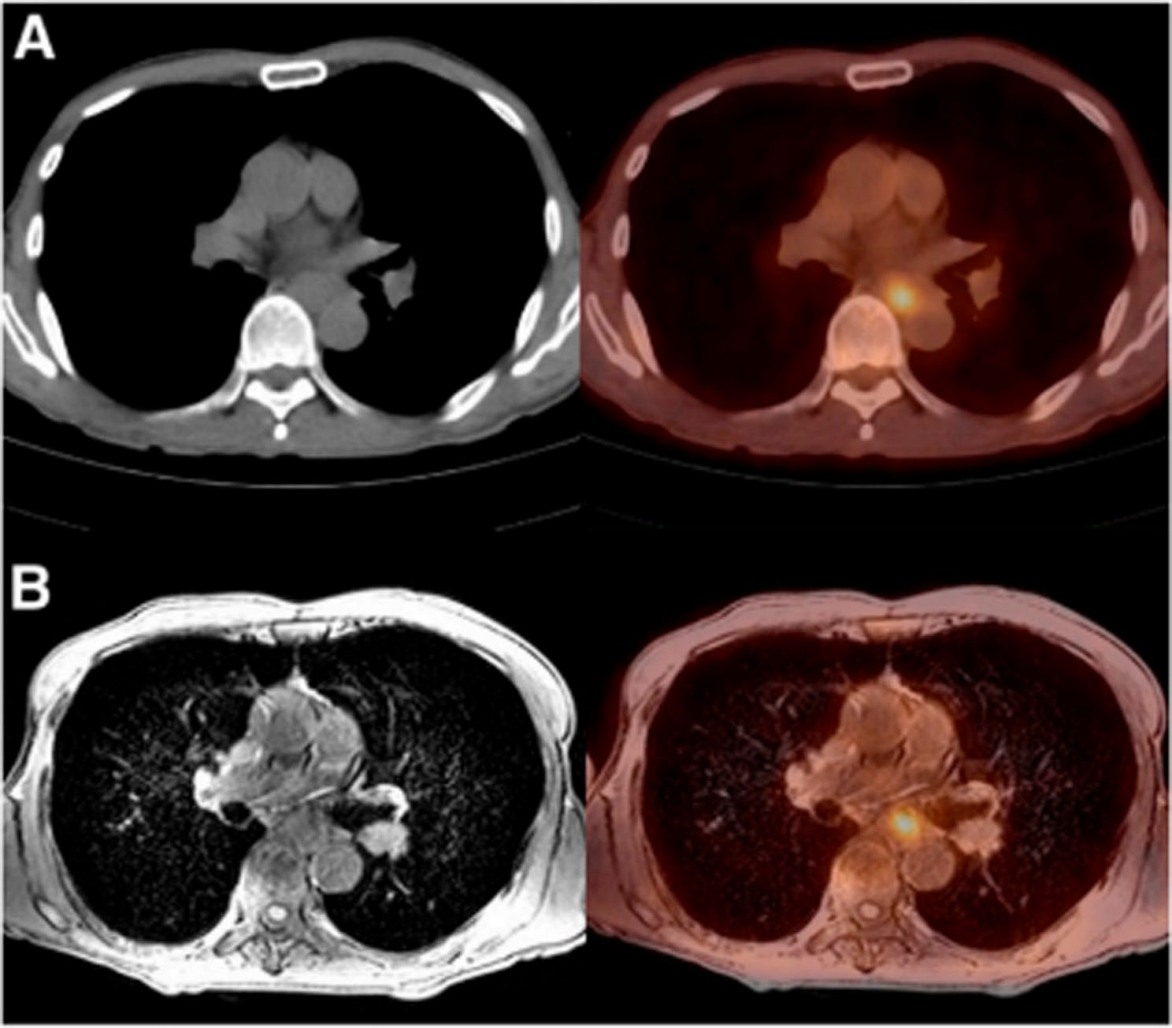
PET/CT (upper row) and PET/MRI (lower row) images of a representative case of esophageal cancer (a 63-year-old man). PET/MRI provides a higher quality image and better visibility than PET/CT [20]
